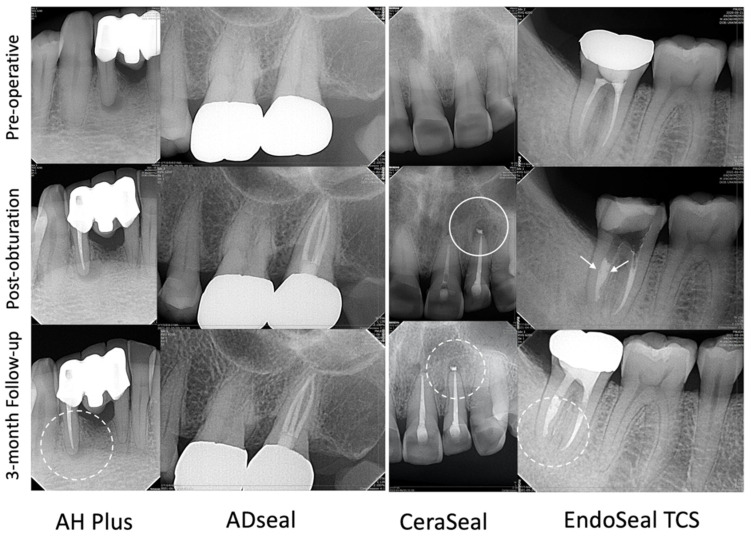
Representative cases obturated with 4 sealers used in this study. Radiographs of pre-operative, post-obturation, and 3-month follow-up from above to bottom. Dot circles show healing or healed apical lesion on 3-month recall. A case with CeraSeal shows sealer extrusion (full circle). A case with EndoSeal shows minimal void (white arrows, scored as 1) in the obturation.
